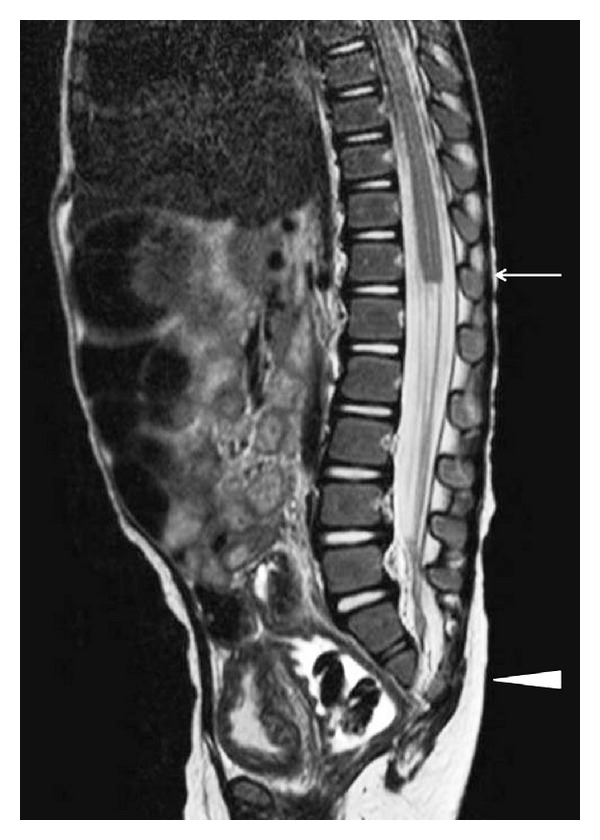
MRI showed the absence of the sacral bone under S2 and distal lumbar vertebrae (arrowhead). The level of the spinal cord terminus was situated as T-12 (arrow).
